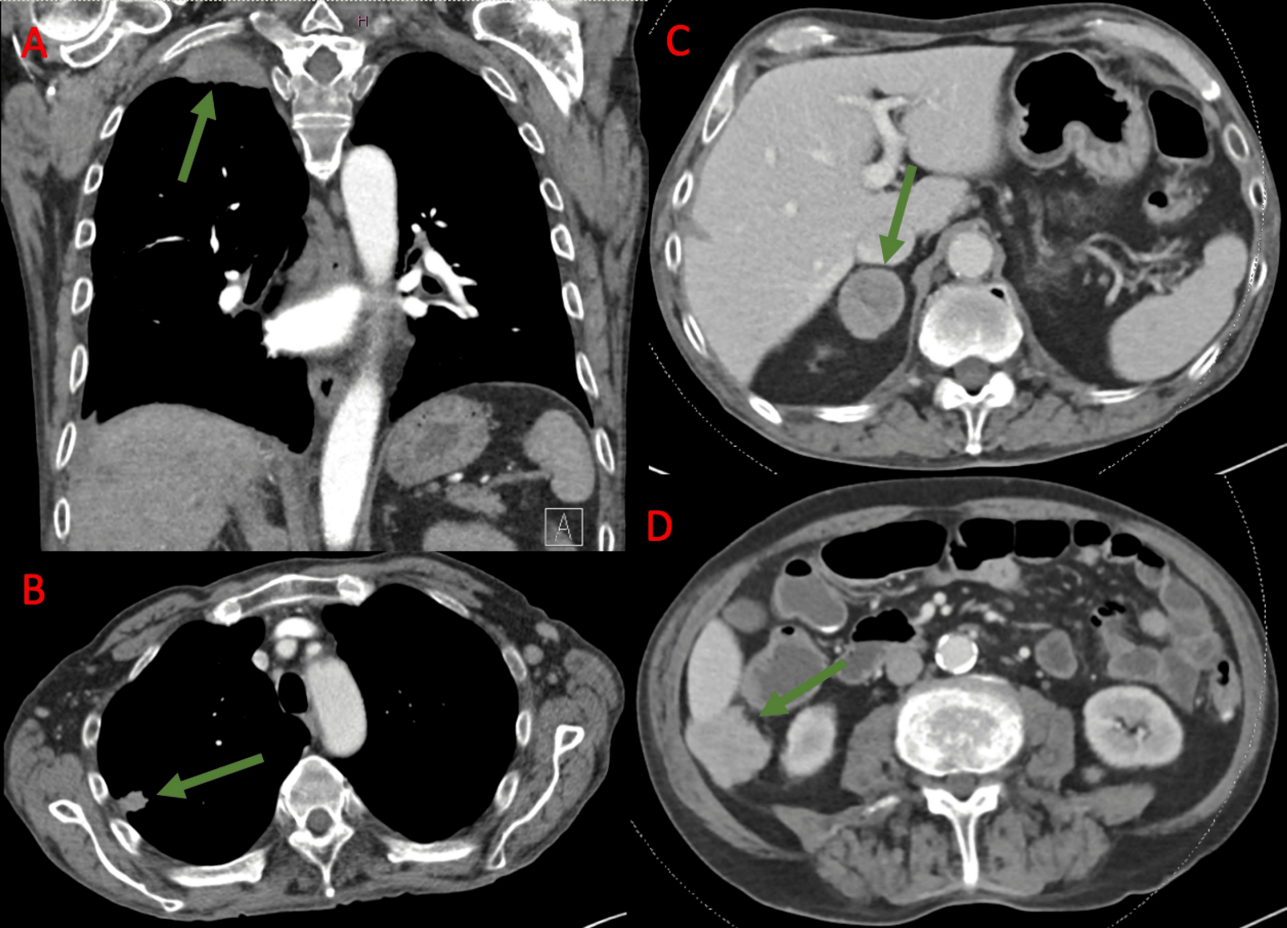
Target lesions prior to initiation of immune checkpoint inhibitor therapy, demonstrated on computed tomography. (A) Apical lung mass measuring 4.6 cm. (B) Pulmonary nodule measuring 1.3 cm. (C) Adrenal nodule measuring 3.7 cm. (D) Right peritoneal nodule measuring 3.5 cm. The green arrow points to the lesion of interest in each image.
